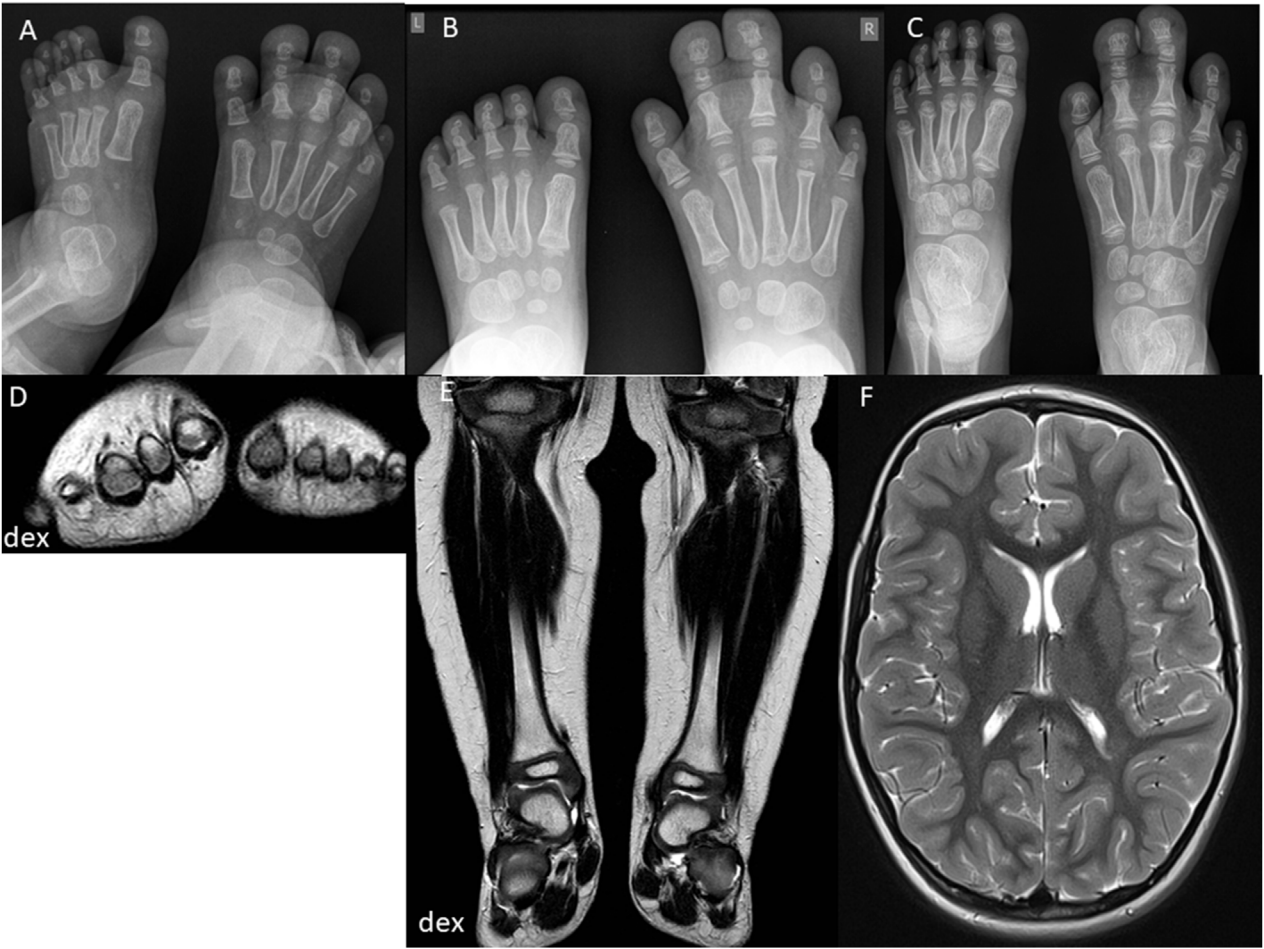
Second case imaging series at different ages. At 1.5 years, X-ray images (A) show increased size and length of metatarsal bones II-IV and toe phalanges II-IV on the right side, with syndactyly of the second and third toes. MRI (D) reveals enlarged metatarsal bones II-IV and increased thickness of plantar and dorsal soft tissues on the right foot. Coronal MRI (E) shows symmetric legs and heels with equal tibial lengths and consistent soft tissue thickness. At 2.5 years, X-ray images (B) indicate proportional growth in both feet, with consistent enlargement and elongation of metatarsal bones II-IV and toe phalanges II-IV on the right side, advanced bone age, and persistent syndactyly of the second and third toes. At 4 years, X-ray images (C) reveal further growth and advanced bone age in metatarsal bones and toes II-IV on the right side, with persistent syndactyly. MRI (F) shows normal and symmetrical cerebral structures and symmetrical development of other body parts without signs of malignancy.
